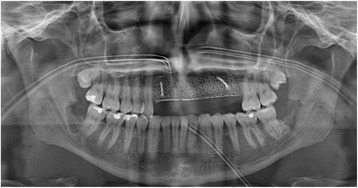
Orthopantomogram after augmentation
